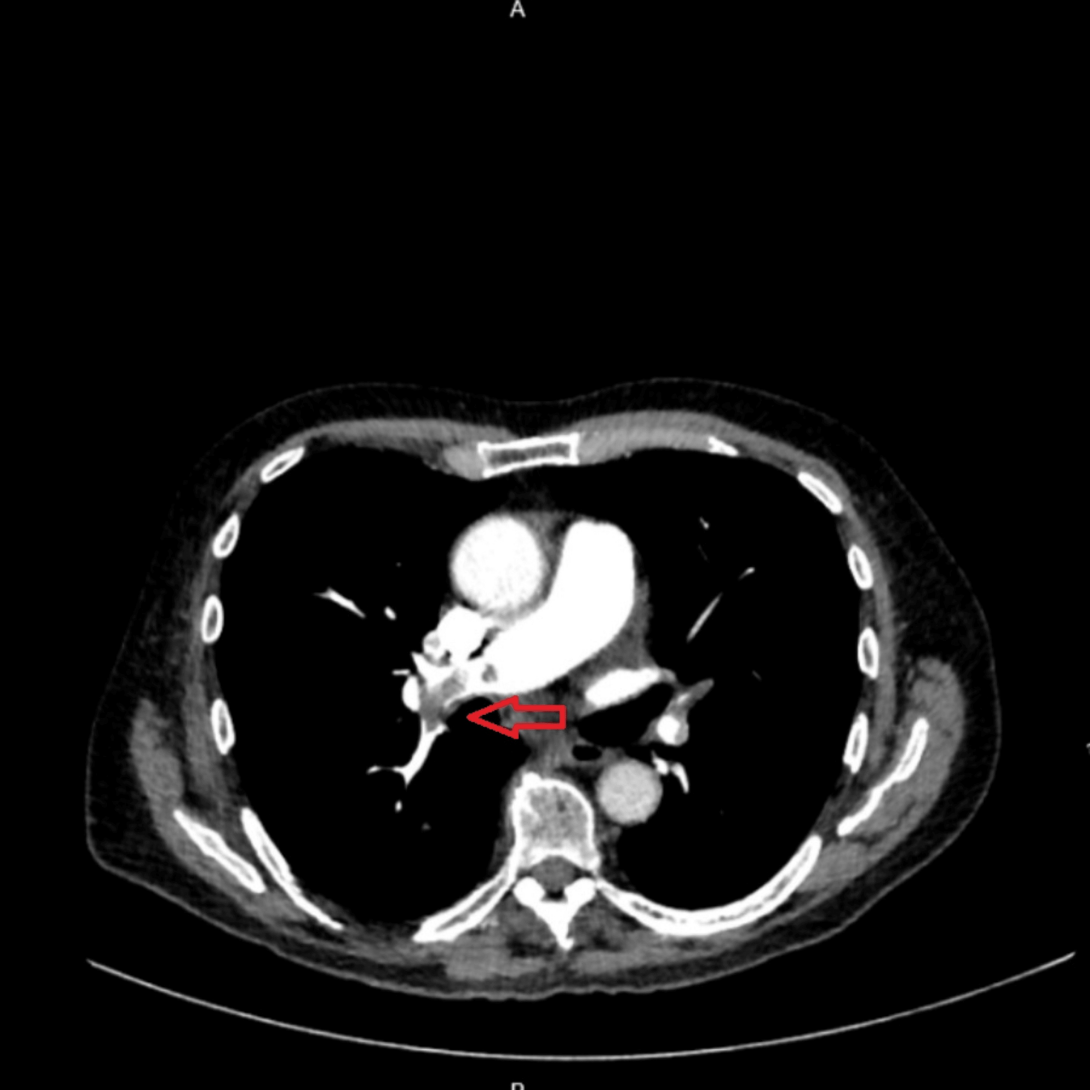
CT pulmonary angiogram showing embolism in the right pulmonary artery
